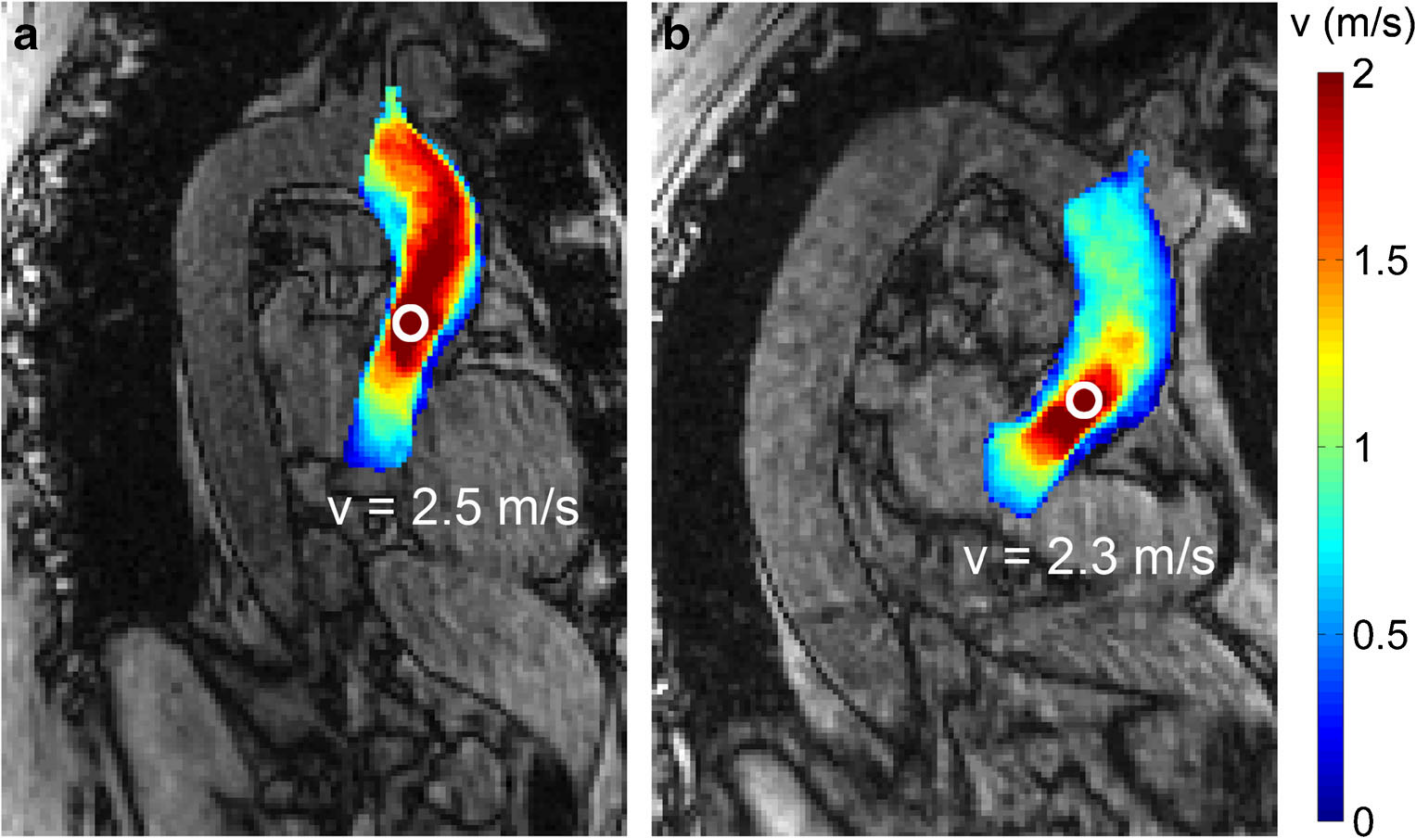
Example of velocity as measured with 4D flow MRI in the ascending aorta in a patient with (a) a stented prosthesis and (b) a stentless prosthesis. 0 = centre of peak velocity
